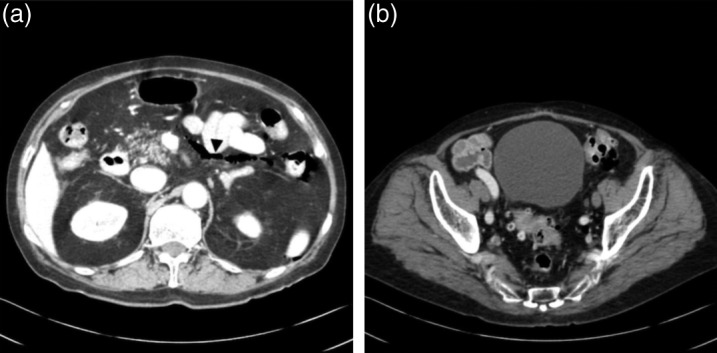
(a) Enhanced CT image of the patient's abdomen showing free air around the sigmoid colon, transverse colon, and descending colon (arrowhead). (b) Enhanced CT image of the patient's abdomen showing numerous sigmoid colon diverticula with stercoroma in the diverticulum.
